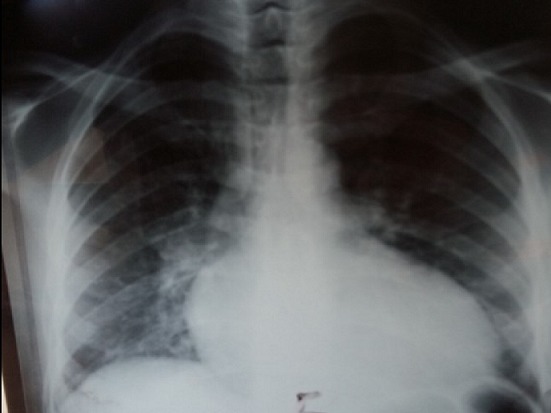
Radiographie pulmonaire de face montrant une cardiomégalie par allongement de l’arc inférieur gauche, un débord droit et une surcharge hilaire bilatérale
